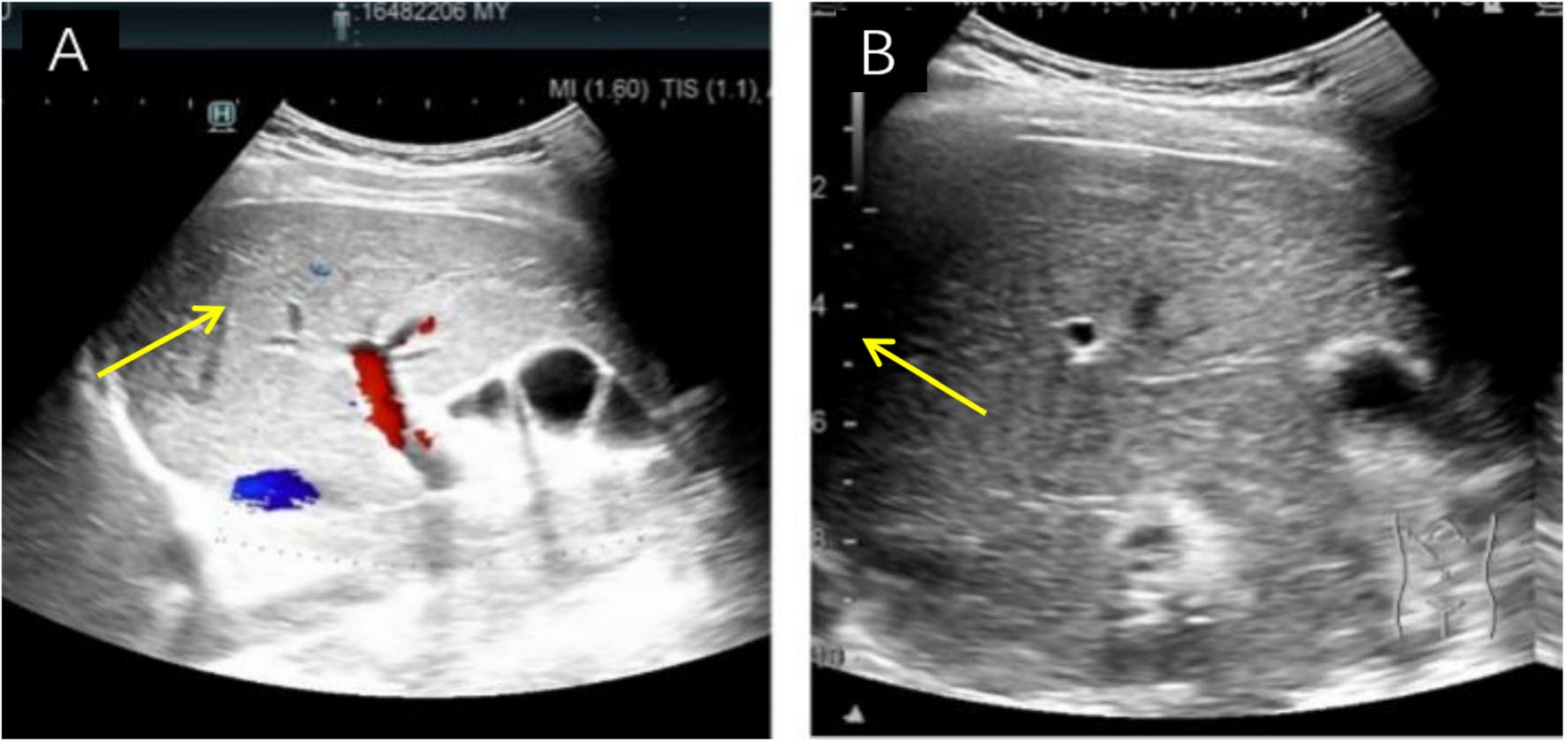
Ultrasound images of the liver. A Transection of the right branch of the intercostal portal vein; B lower oblique section of the right costal margin. Ultrasound of the liver revealed increased hepatic parenchymal echogenicity. The liver volume is normal
